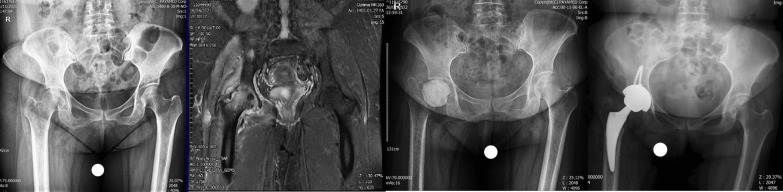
a Case three, preoperative X-ray, right side involvement. b MRI of the hips showing right side joint destruction. c Post-op radiography after first stage of arthroplasty. d Post-op radiography after second stage of THA
